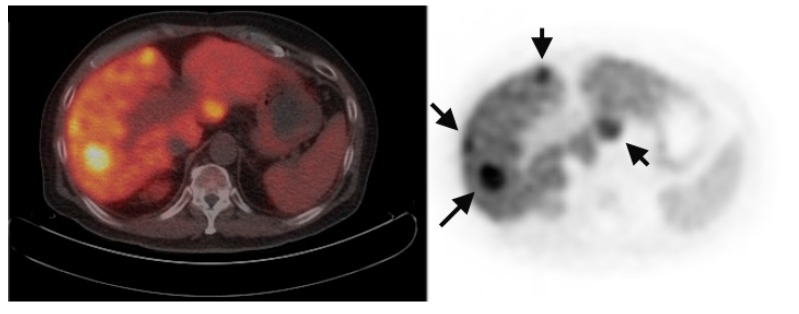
Multifocal recurrent hepatocellular carcinoma (HCC). Corresponding positron emission tomography (PET)/computed tomography (CT) (left) and PET (right) images demonstrate multiple foci of increased fluorocholine (FCh) uptake in the liver (*arrows*) ranging in diameter from 7 to 30 mm.
